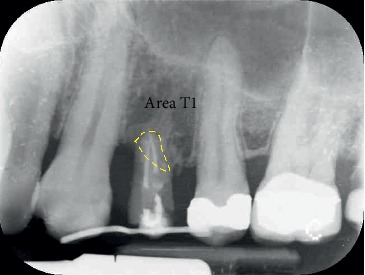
Tooth 2.4: X-ray at T1: the area selected (yellow contour) represents the part of the root inside the bone after 12 weeks of stabilization. The difference between area T0 and area T1 gives the value of bone gain in mm^2^.
